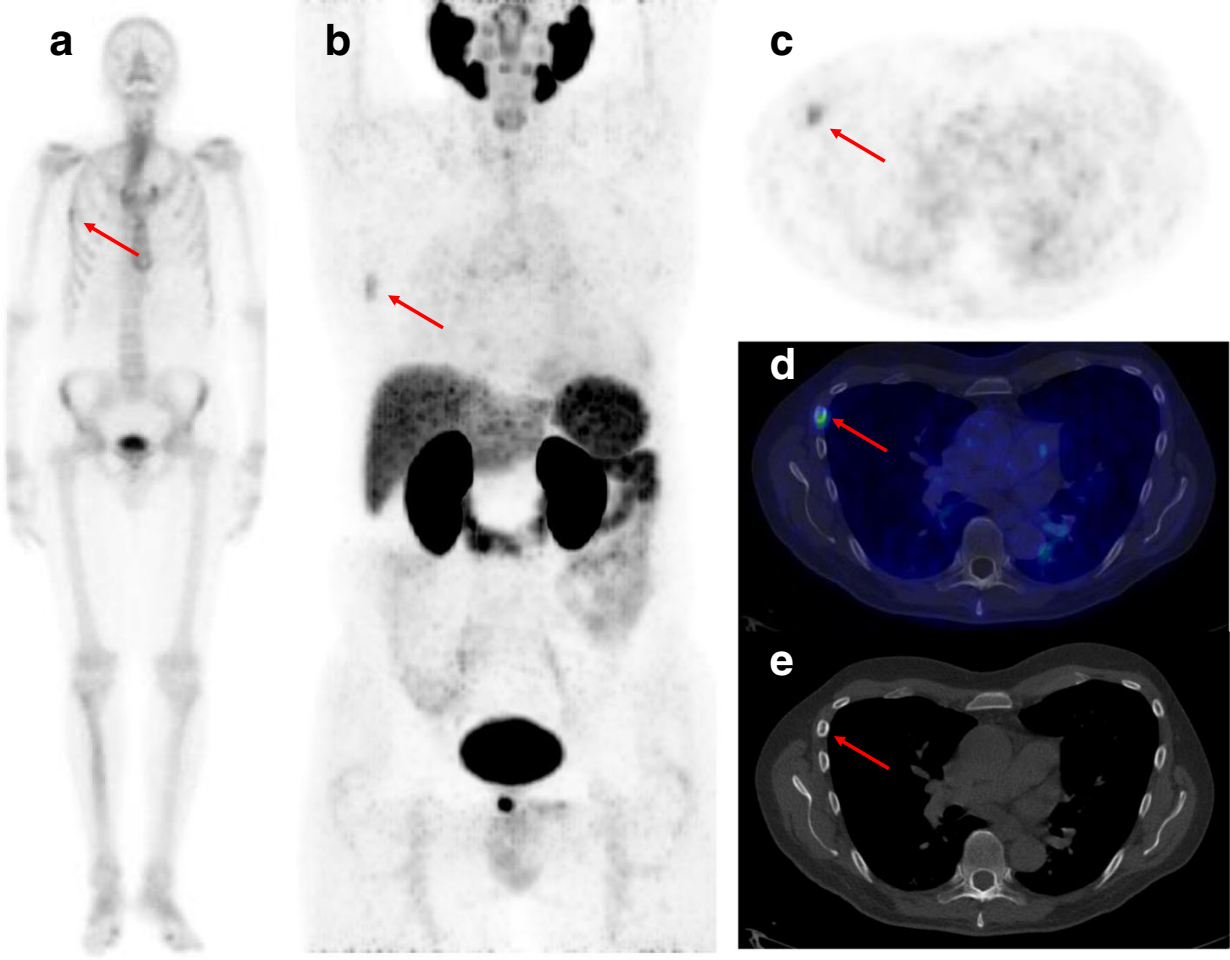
Example of a patient (PSA 6 ng/mL, Gleason score 7, T2b) with four equivocal lesions according to BS (shown in anterior projection) (a) including a lesion in the fourth right rib indicated by the arrow. According to the ^68^Ga-PSMA-11 PET/CT, the lesion was considered metastatic as shown by the ^68^Ga-PSMA-11 MIP (b) with the corresponding axial ^68^Ga-PSMA-11 PET (c), PET/CT fusion (d), and a slight sclerotic lesion in the axial CT image (e). Except for the high ^68^Ga-PSMA-11 uptake in the prostate, no other suspicious lesions were observed. The patient underwent a CT-guided biopsy that showed benign findings, and then the patient had a radical prostatectomy without any systemic treatment. The PSA dropped to < 0.1 ng/mL after prostatectomy and remained so for at least 12 months
